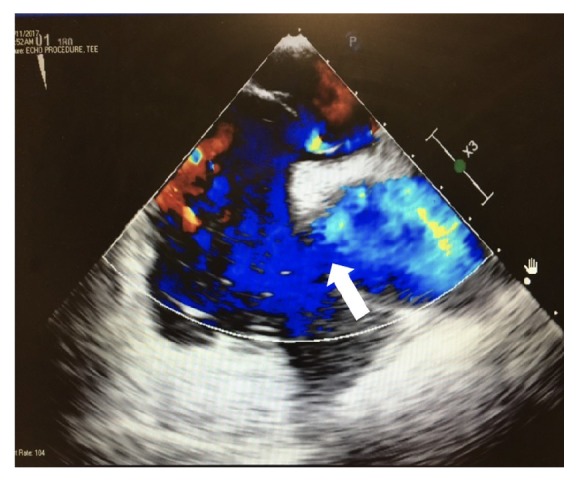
Transesophageal echocardiogram showing significant R to L shunt (white arrow).
